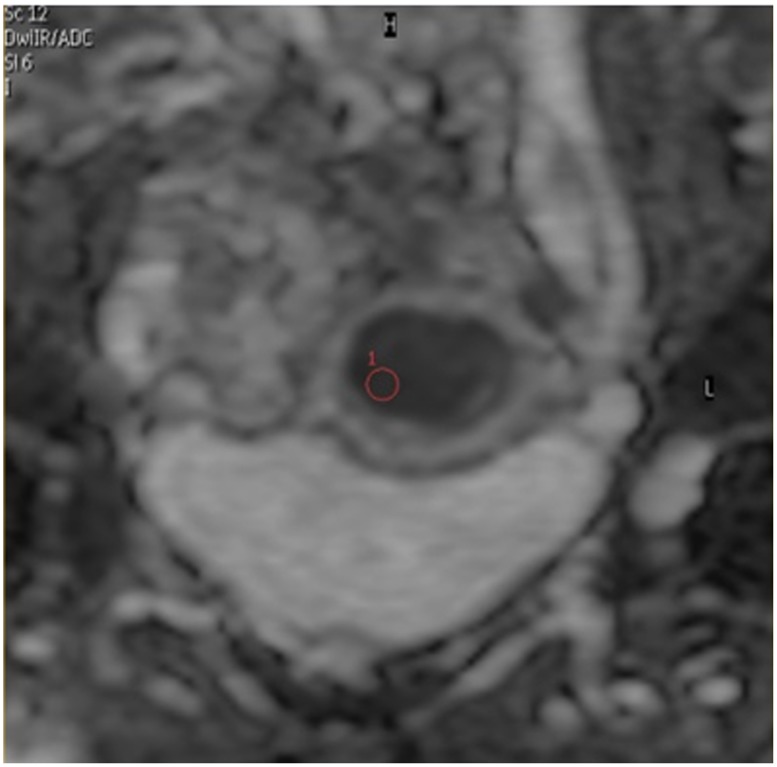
Apparent diffusion coefficient (ADC) analyses of endometrial lesions MRI identified a endometrial carcinoma invading the endometrial cavity with intermediate signal intensity on axial oblique T2-weighted imaging (A) and high signal intensity on diffusion weighted imaging (DWI) (B). A region of interest (59.3 mm^2^) was manually defined within the smallest ADC area of the endometrial lesion on a DW-ADC image (C).
